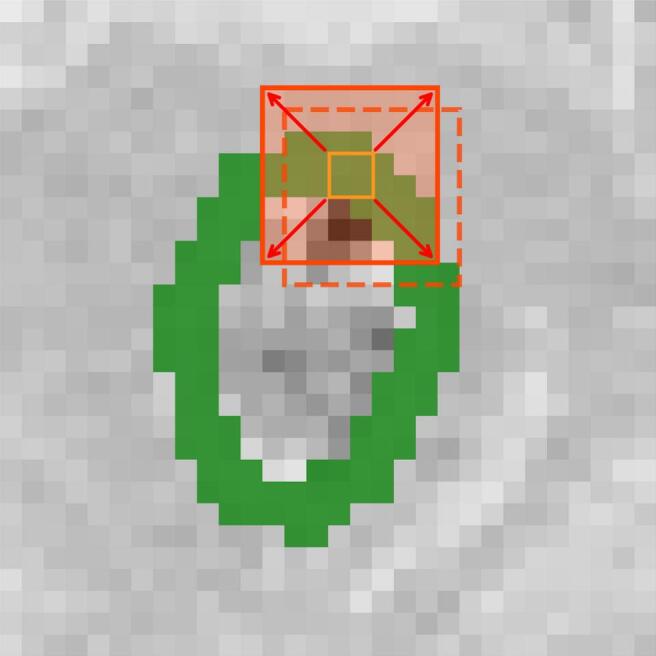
Example of rectal wall subimages extraction using planning CT. (a) The manually delineated rectum (green), (b) the resulting 2-pixel-wide rectal wall region of interest (green) after inward expansion, with surrounding 8x8 pixel^2^ subimages (orange squares) extracted at 1-pixel intervals by expanding the ROI anteriorly and posteriorly by 3 pixels. (For interpretation of the references to colour in this figure legend, the reader is referred to the web version of this article.)
